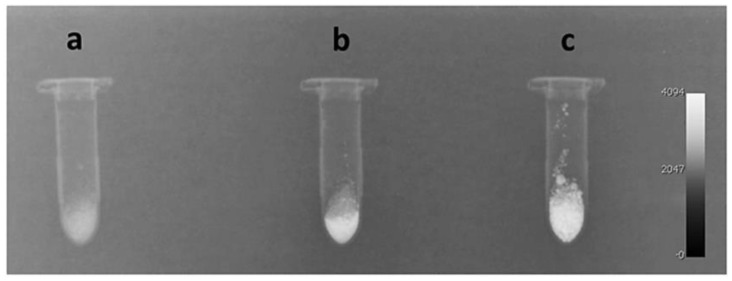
X-ray image showing the radiopacity comparison of a SiO_2_–CaO–P_2_O_5_–ZrO_2_ gel-glass powder: (a) without Zr; (b) doped with 7 wt.% Zr; (c) doped with 15 wt.% Zr [146].
